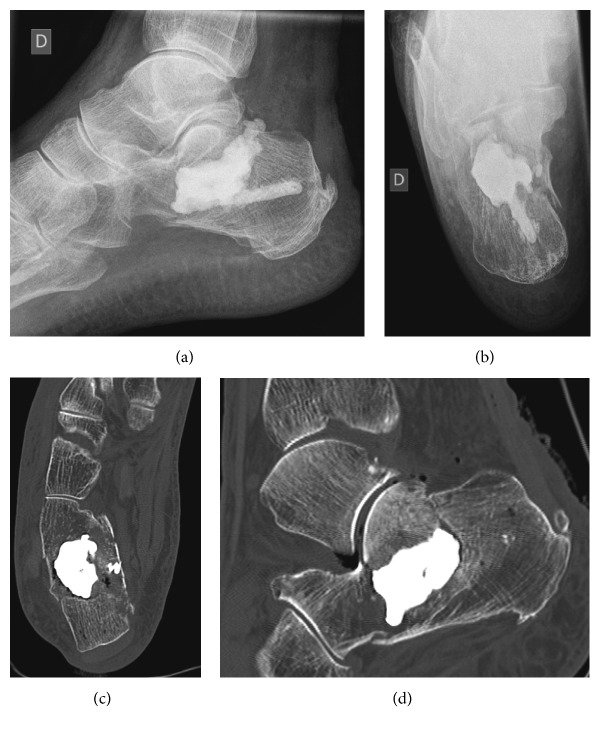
On the upper part, postoperative radiographs showed a Boehler”s angle from 33 degrees. On the lower part, postoperative CT scan showed satisfying alignment of the bone fragments, with presence of a discrete diastasis at the level of the posterior subtalar fracture, but without irregularity of the articular surface.
